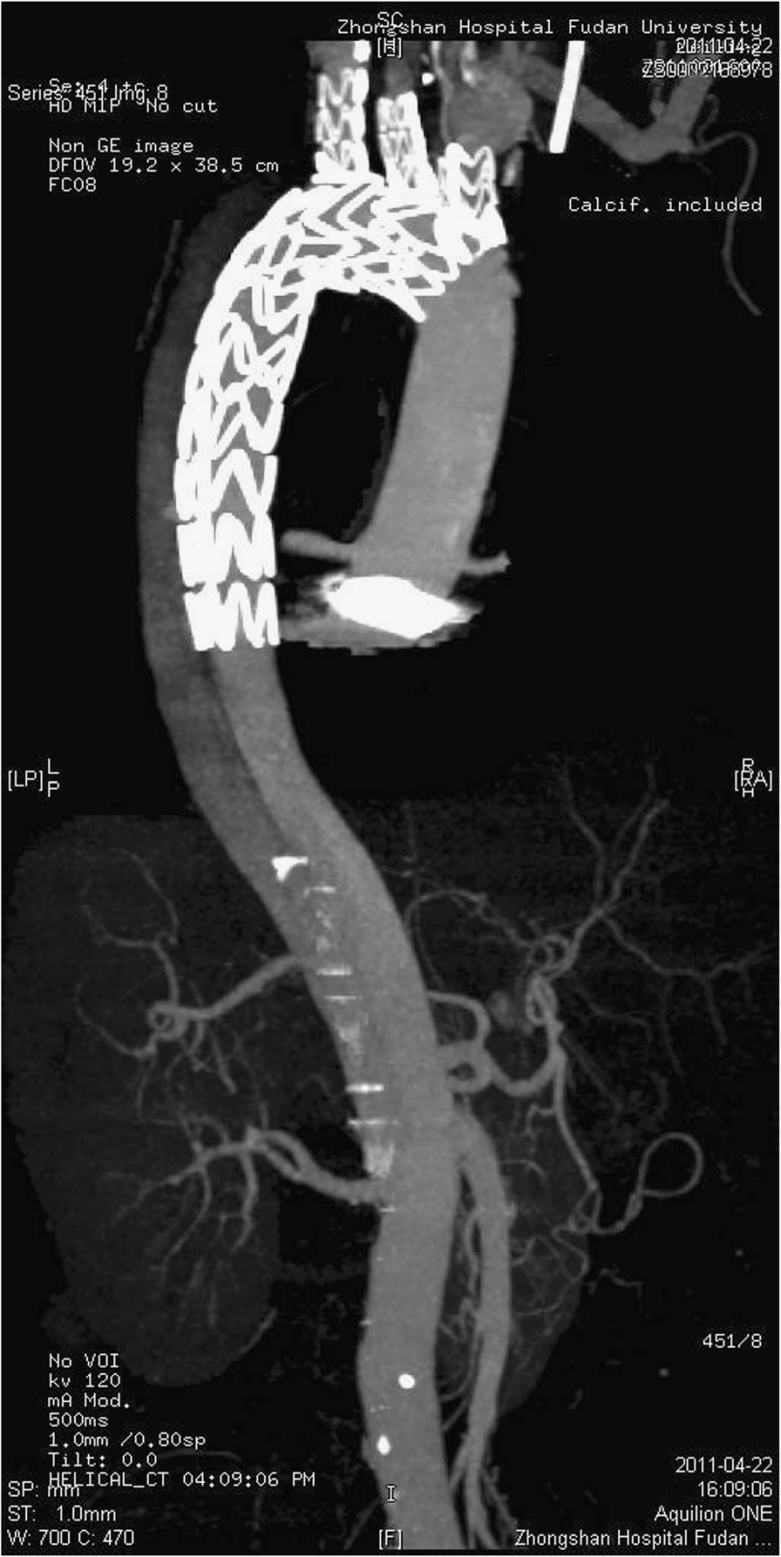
Angio CT Scan of the patient after surgical intervention shows that the true channel in the descending aorta was resumed, and the false channel was closed with thrombus.
